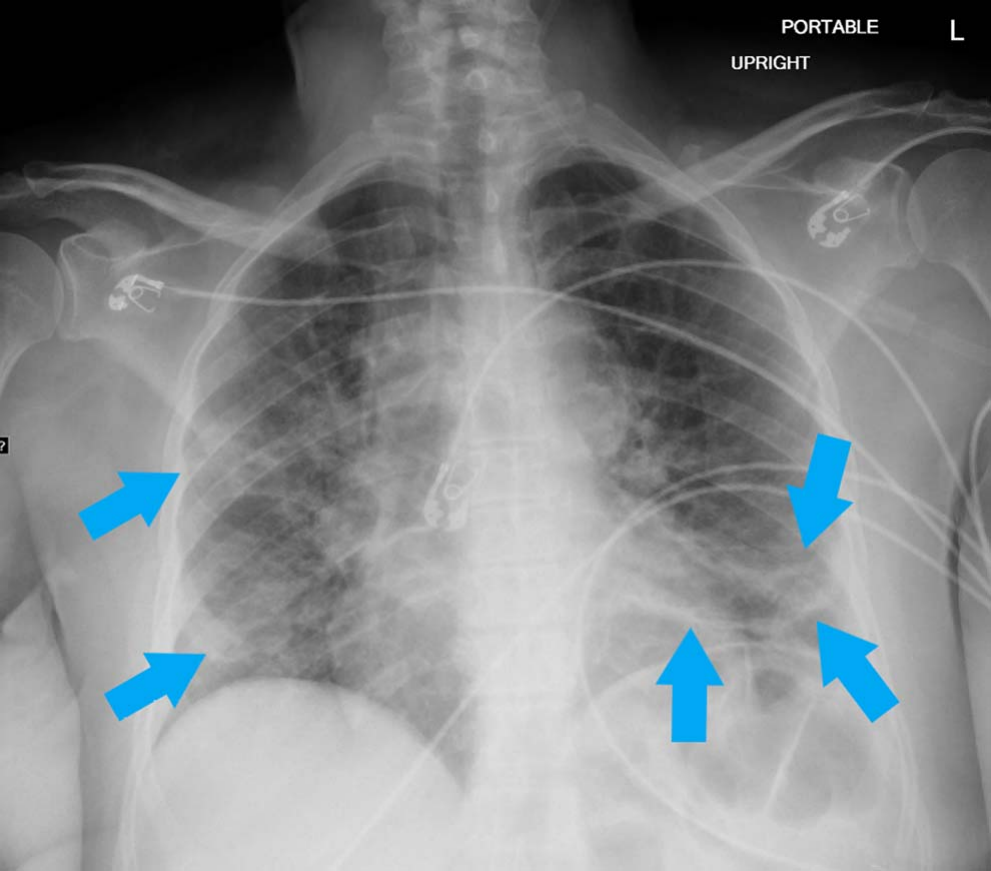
Portable upright anteroposterior radiograph of a 65-year-old female shows characteristic bilateral confluent, bandlike (arrows) consolidative opacity in a peripheral, mid-to-lower lung zone distribution admixed with patchy airspace opacity.
